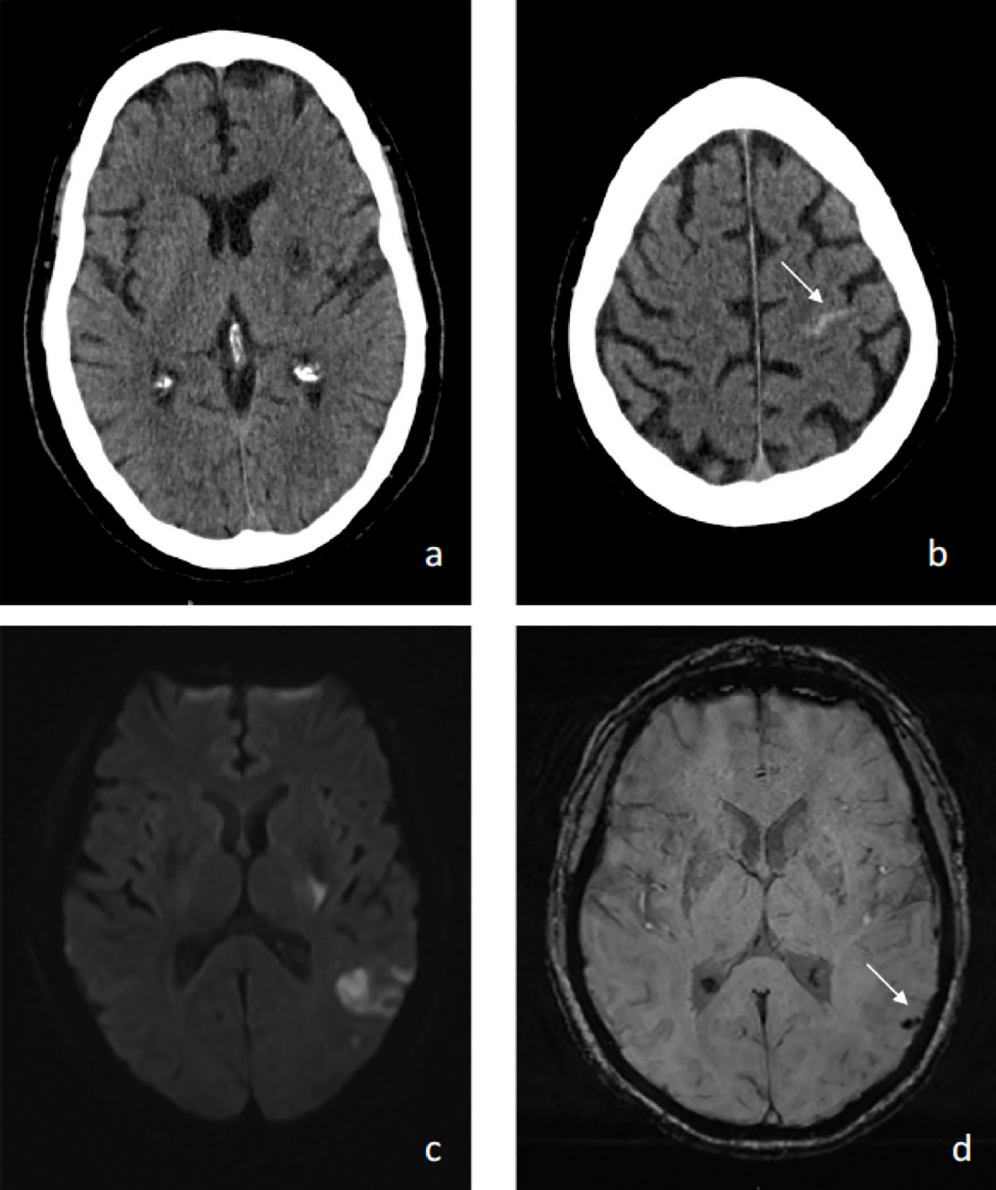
Neuroimaging is shown for the two patients with asymptomatic hemorrhage. Images (a) and (b) are from the 24 hour NCHCT on subject #2, images (c) and (d) are from the follow up MRI on subject #12. The arrows point to areas of hemorrhage.
